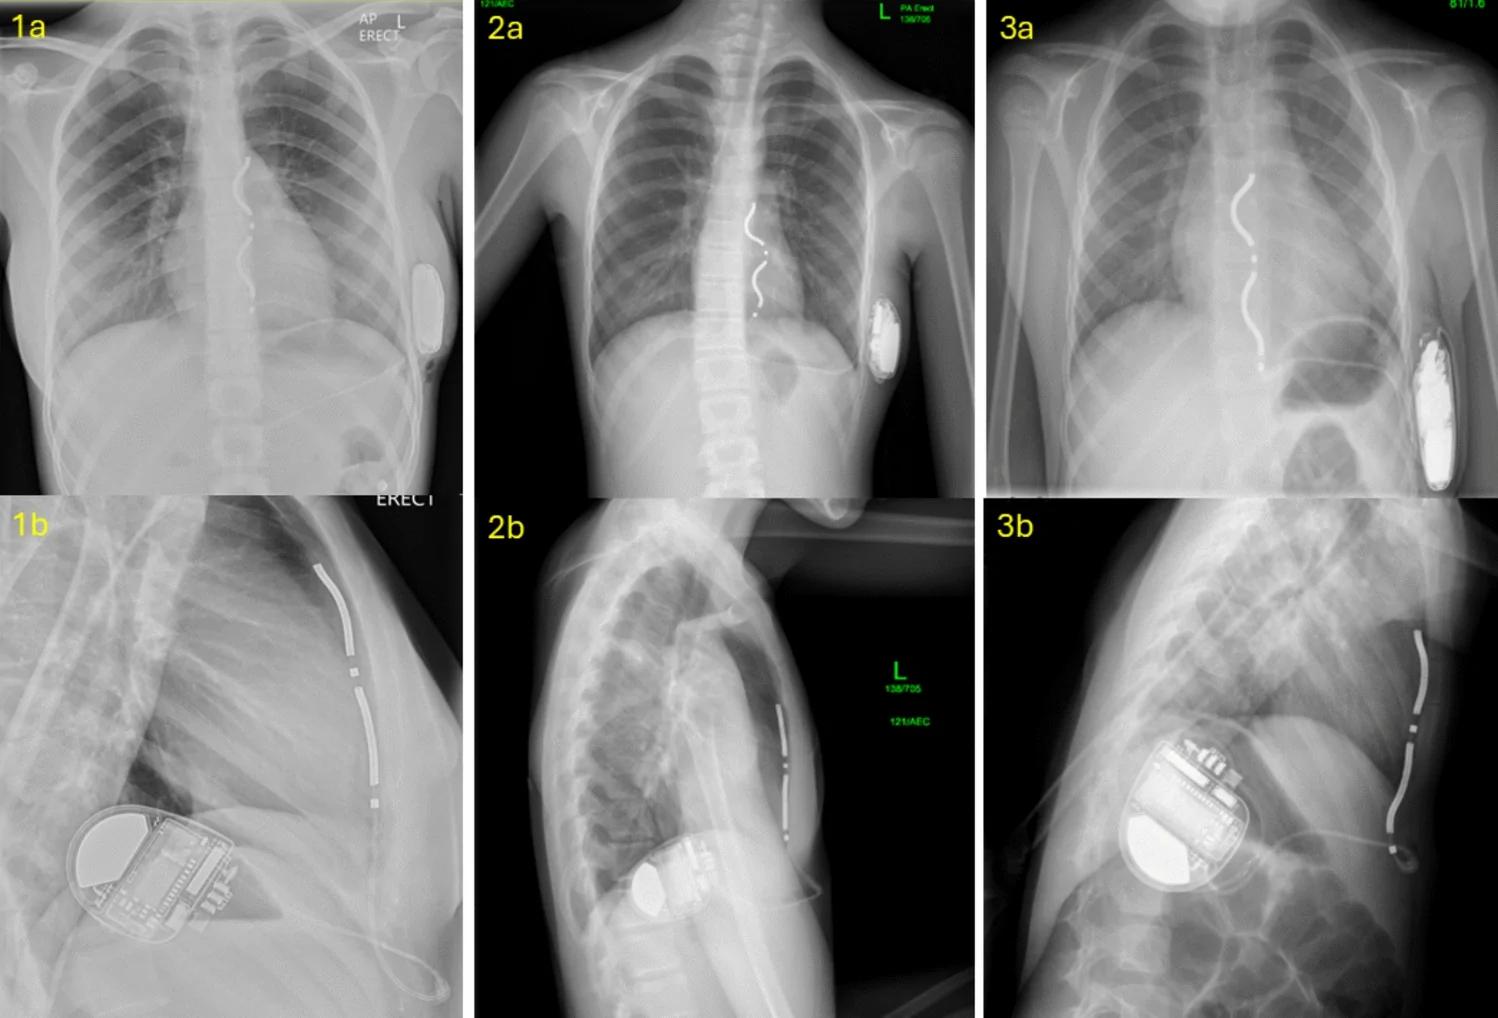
Posteroanterior chest x-rays for case 1 (1a), case 2 (2a) and case 3 (3a). Lateral projection for case 1 (1b), case 2 (2b) and case 3 (3b)
